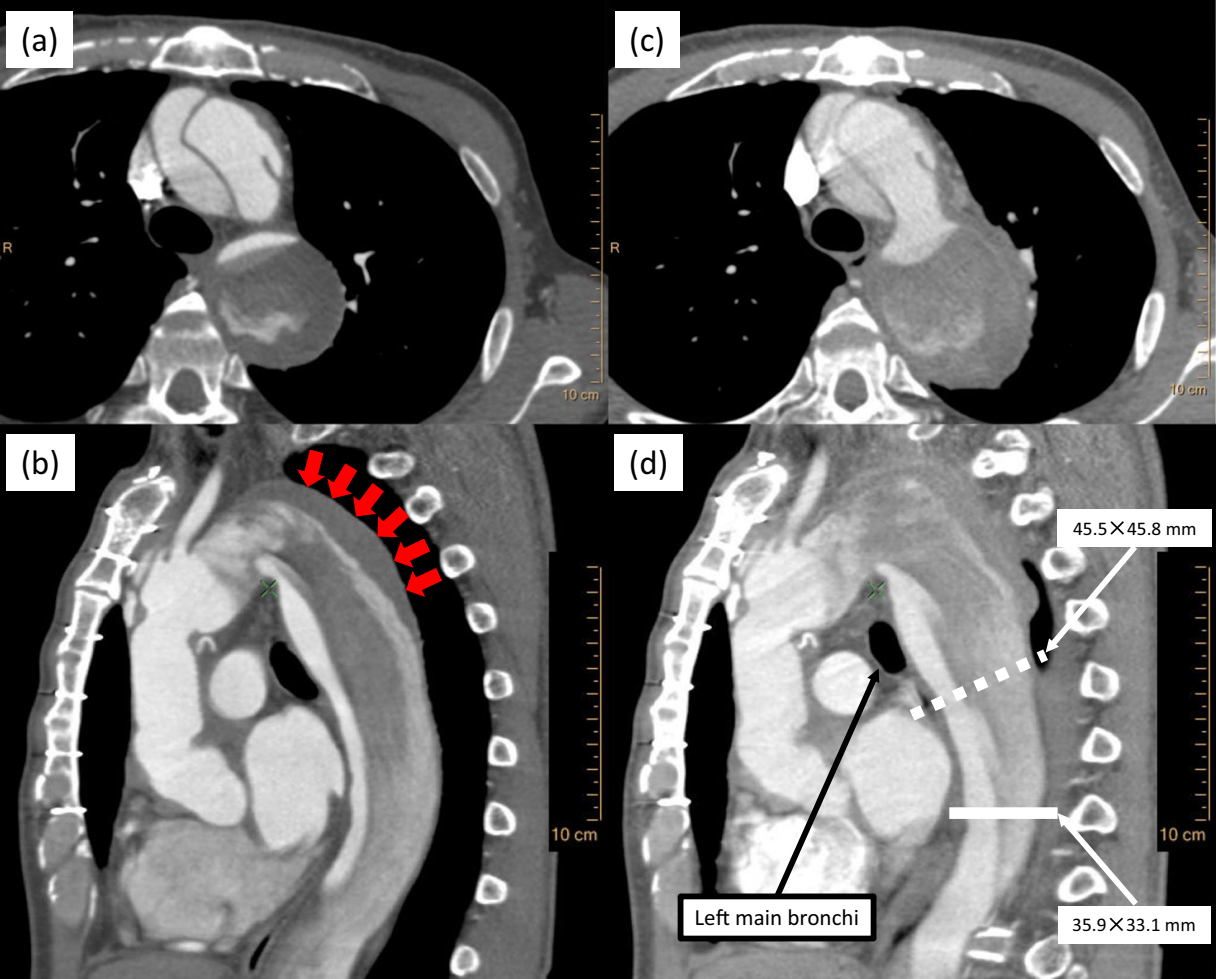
Preoperative computed tomography. The distal aortic arch expanded rapidly within 2 months; at 2 months before admission (a axial, b sagittal), thrombi are seen in the false lumen of the distal aortic arch (red arrows), and at admission (c axial, d sagittal). d White dotted line indicates the estimated distal anastomosis site, while the white solid line defines, where the distal anastomosis was actually performed
